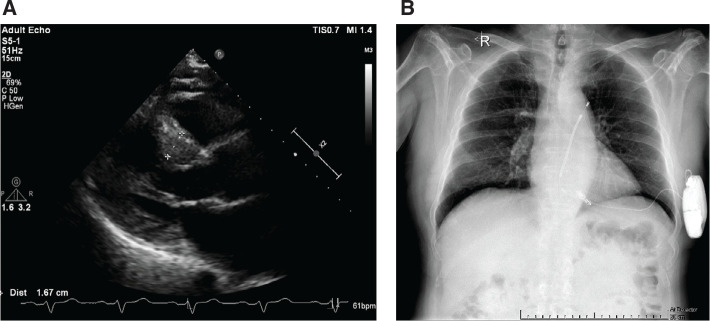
A: An echocardiographic parasternal short-axis view with septal hypertrophy measuring 1.67 cm. B: A chest X-ray after implantation of a leadless pacemaker and subcutaneous defibrillator.
